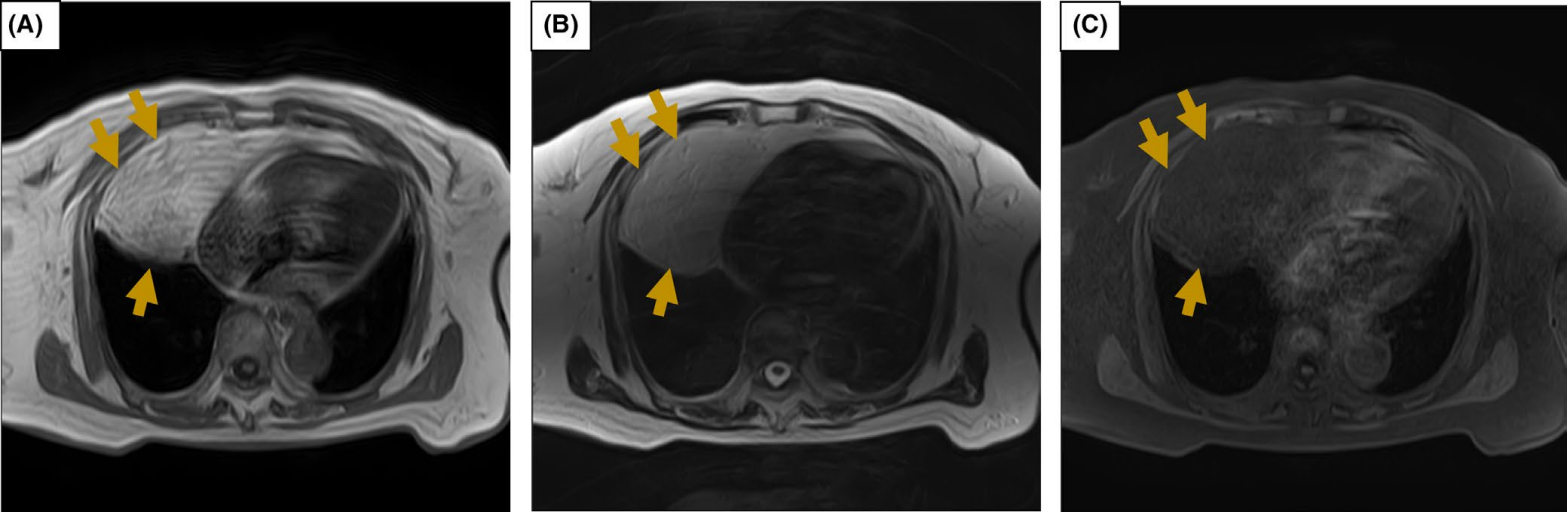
Findings of the recent MRI. (A) T1‐weighted image, (B) T2‐weighted image, (C) Fat suppression technique image. MRI showed a homogenous mass with smooth edge, with the signal intensity reduced by the fat suppression technique (yellow arrow). These findings suggested the diagnosis of mediastinal lipoma without component of liposarcoma
